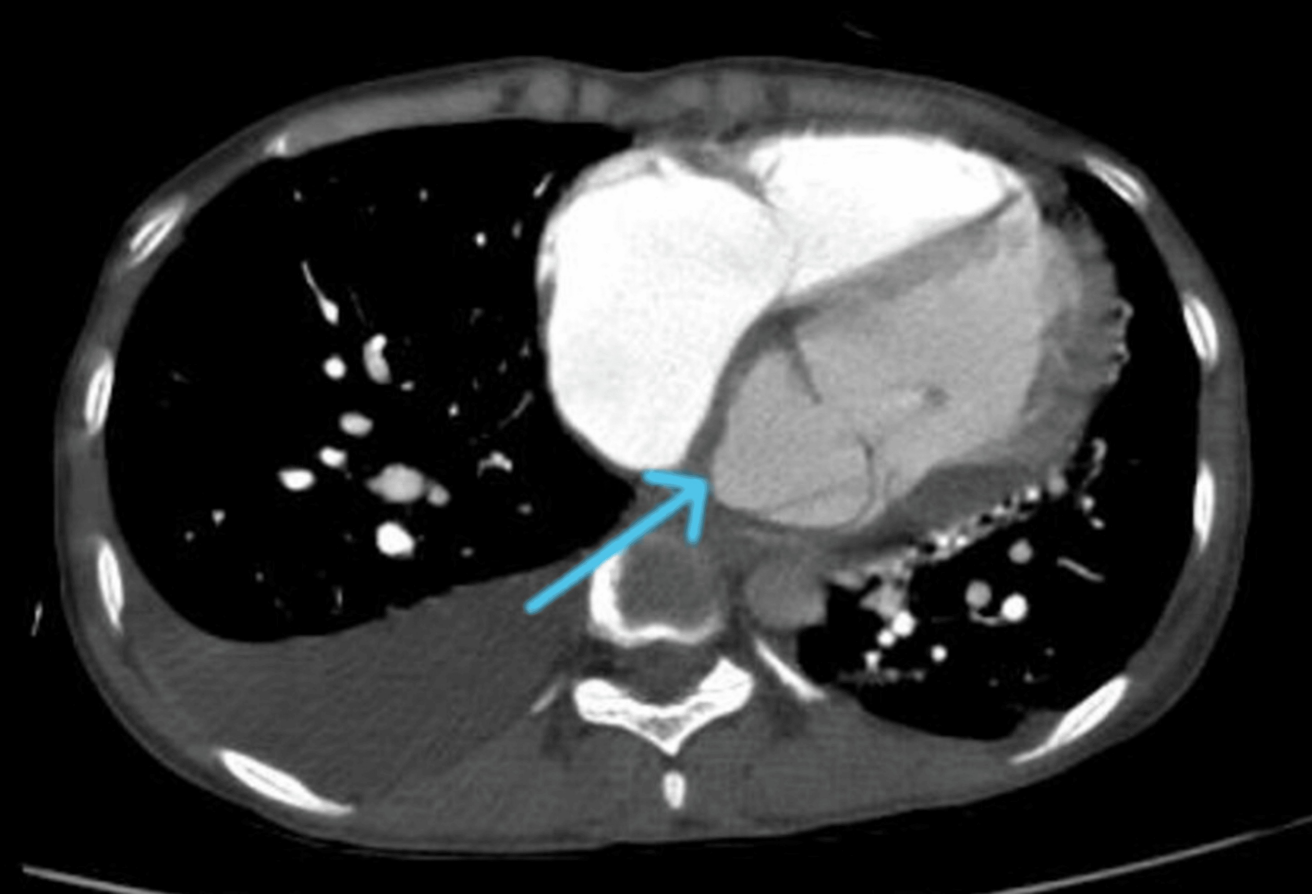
Axial contrast-enhanced cardiac CT image of the chest showing a large 40 × 29 mm pseudo-aneurysm (blue arrow) arising from the basal segments of the left ventricle
